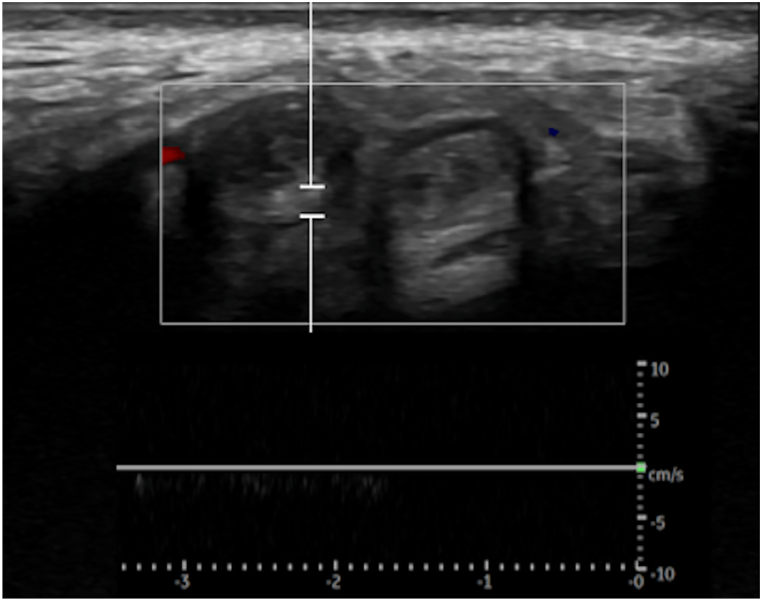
Pulse wave doppler ultrasound image showing hyperechoic blood clots within the dorsal penile vein and the left dorsal penile artery. A lack of flow within the dorsal penile vein is confirmed by the lack of signal.
